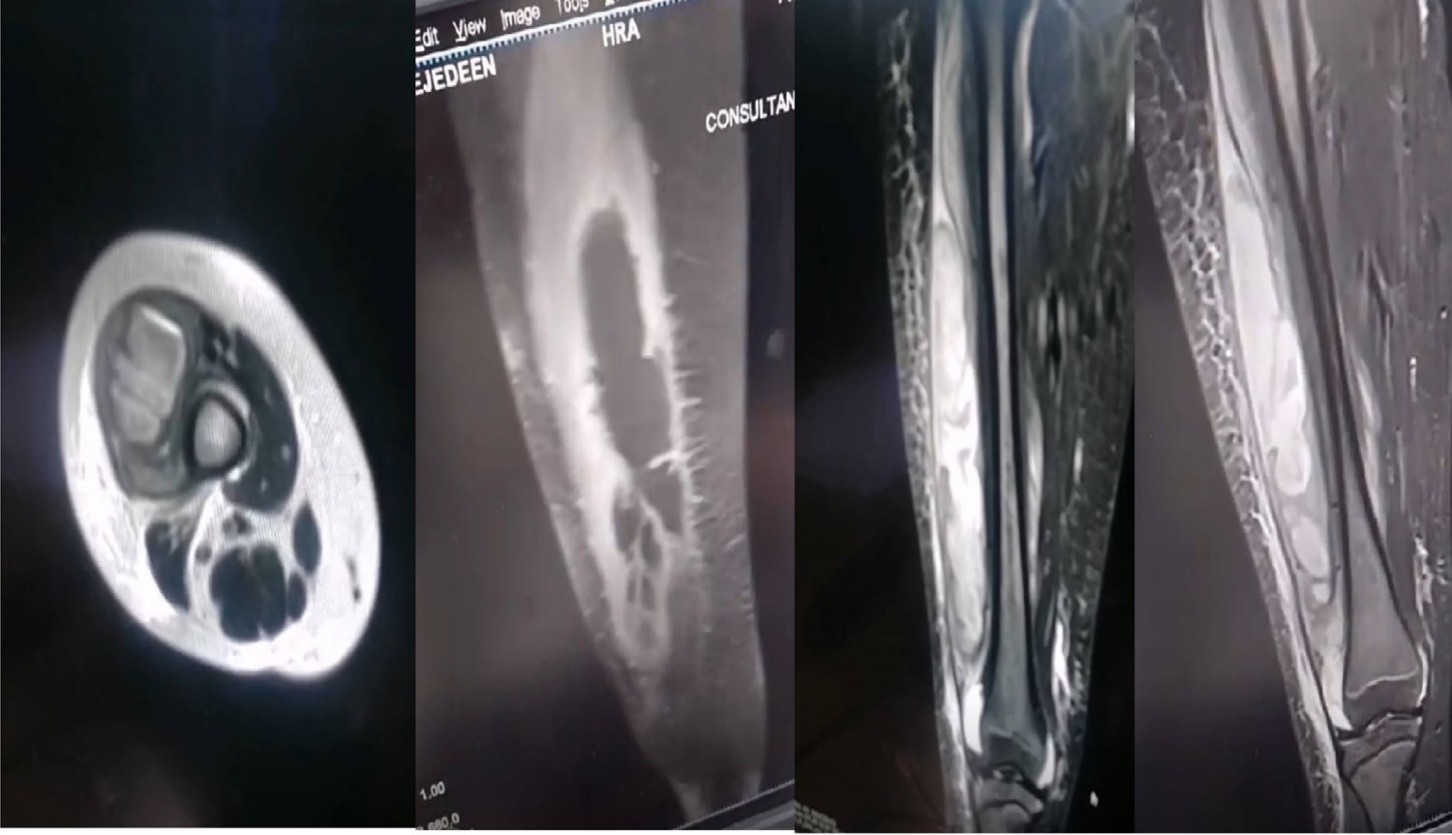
Axial, sagittal and coronal sections of Right thigh MRI showing significant amount of deep Intramuscular collection with no obvious signs of osteomyelitis.
